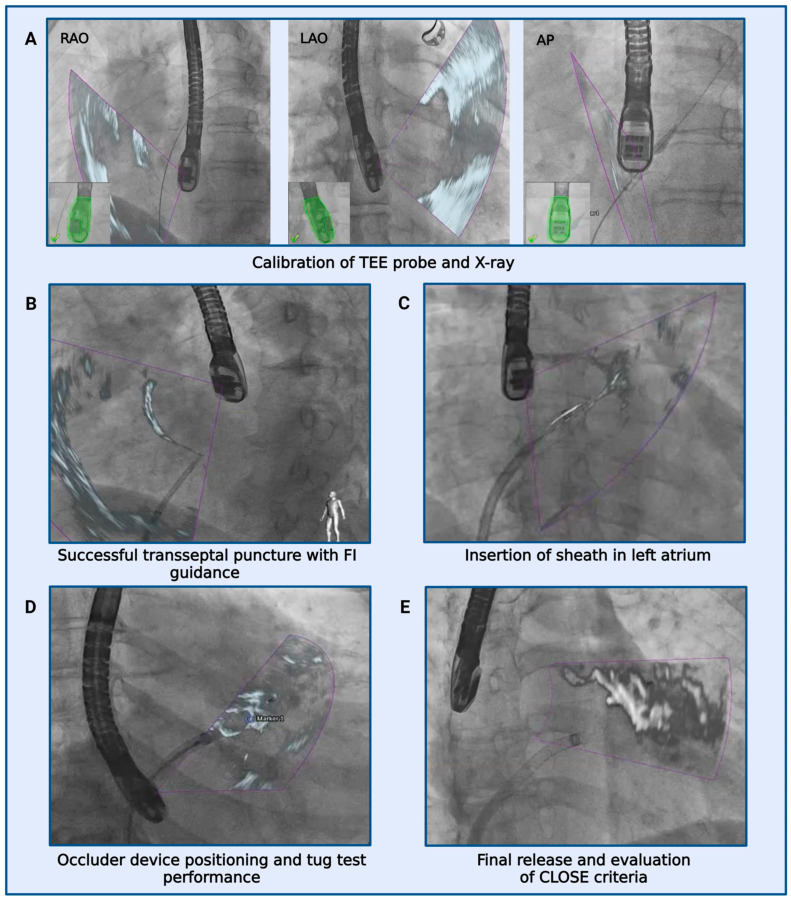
LAAC step by step under FI II guidance. (A): Green color confirmed correct positioning of the TEE probe. (B–E): Purple triangle depict real-time fluoroscopic and echocardiographic overlay.
